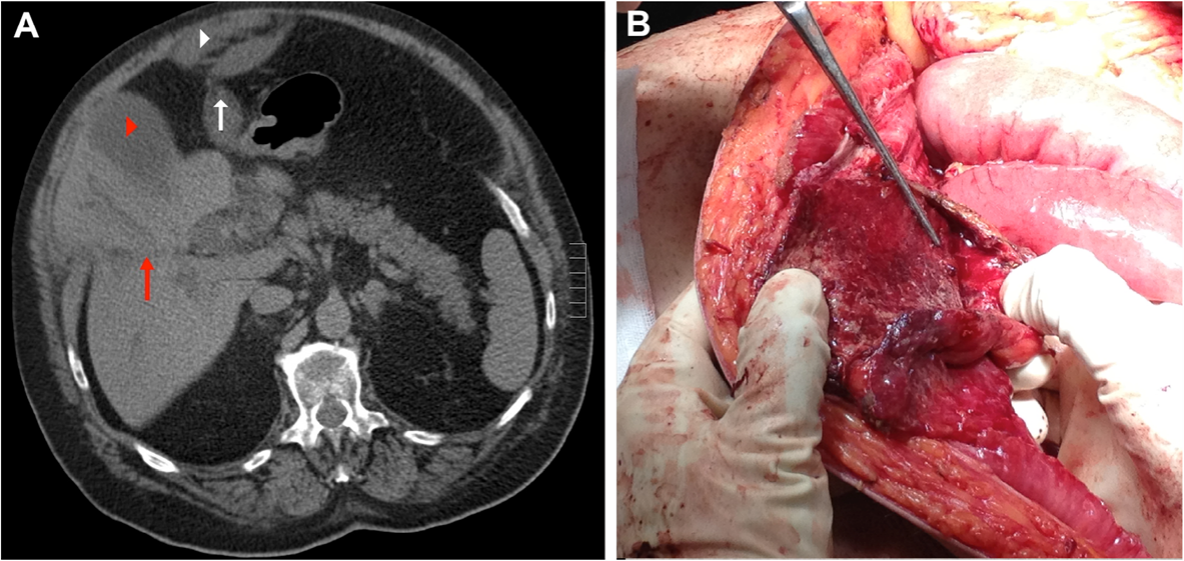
CT abdomen. (A) The enlarged gall bladder (red arrow head) and dilatation of intrahepatic bile ducts (red arrow). A fluid collection is seen tracking from the liver along the path of the falciform ligament (white arrow) to a collection on the anterior abdomen (white arrow head). (B) Operative view of the rind of thickened inflammatory falciform tissue (black arrow) after abscess drainage.
